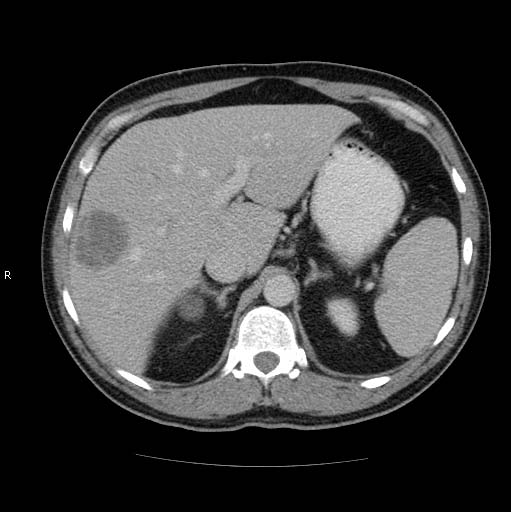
Computed tomography of the abdomen showing a mildly enhancing peripheral hypodense lesion in liver. Scan taken during the initial admission.
